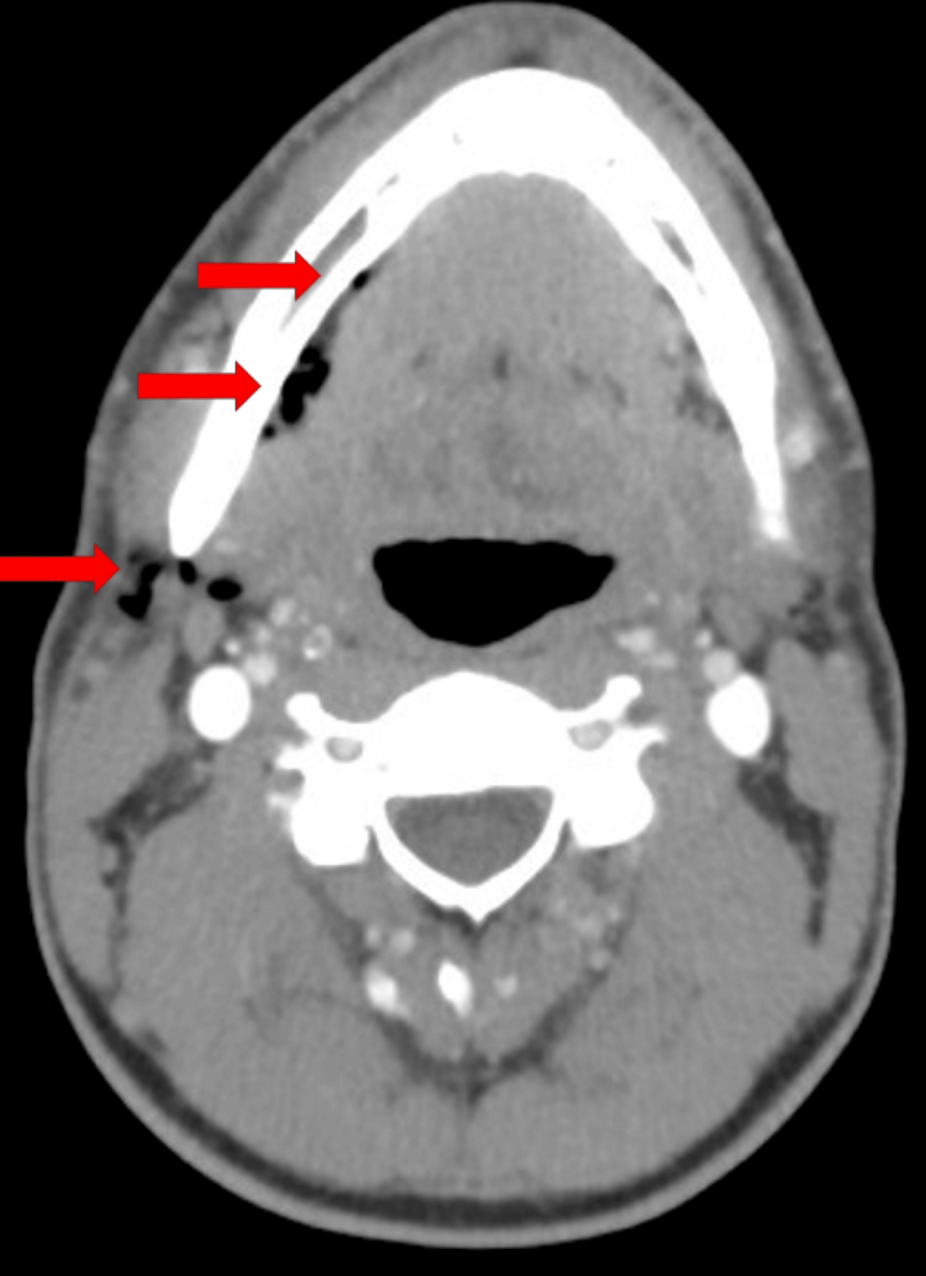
Computed Tomography: Axial/Transverse View Red arrows indicate areas of subcutaneous emphysema.
